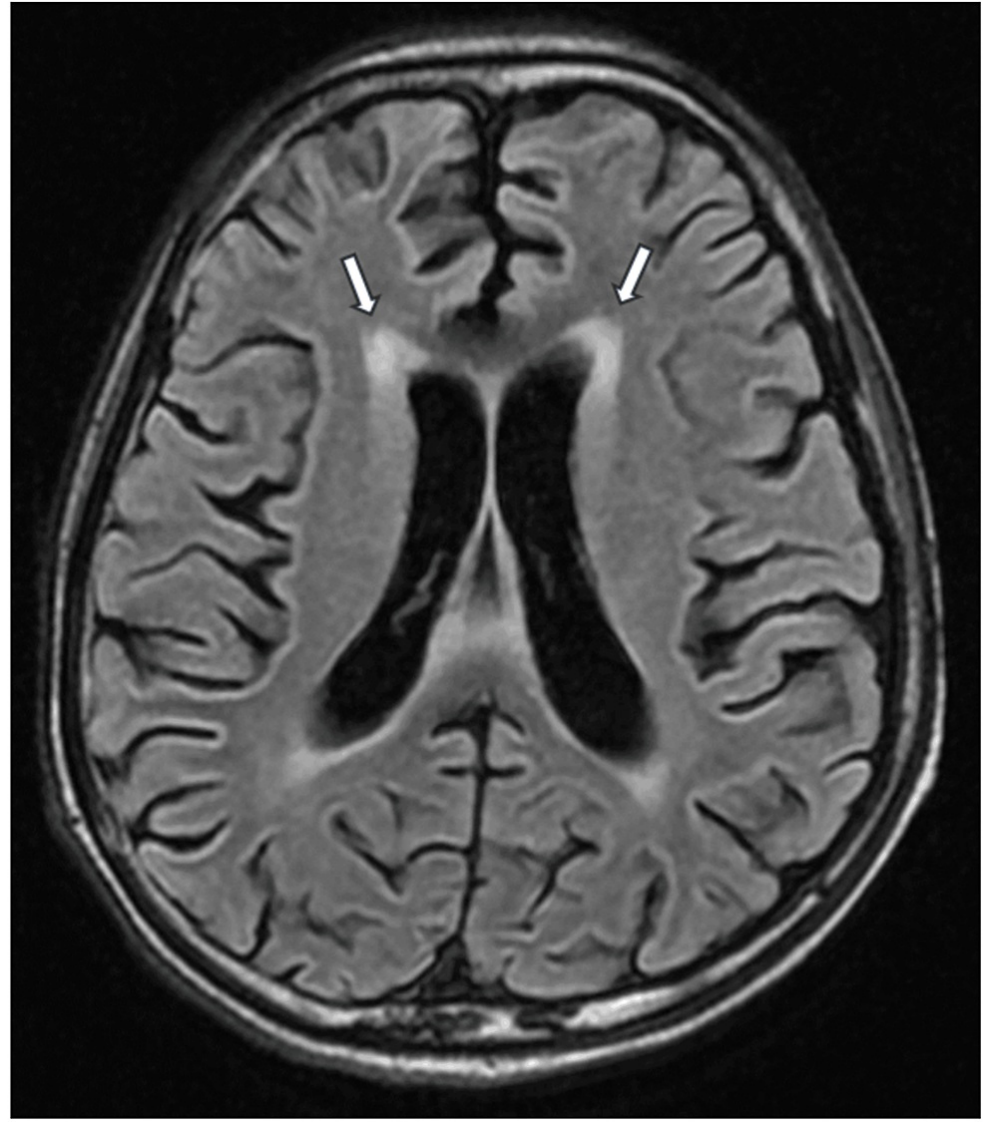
Flair MRI brain (axial section) reveals diffuse periventricular white matter hyperintensities (white arrow) MRI: magnetic resonance imaging
